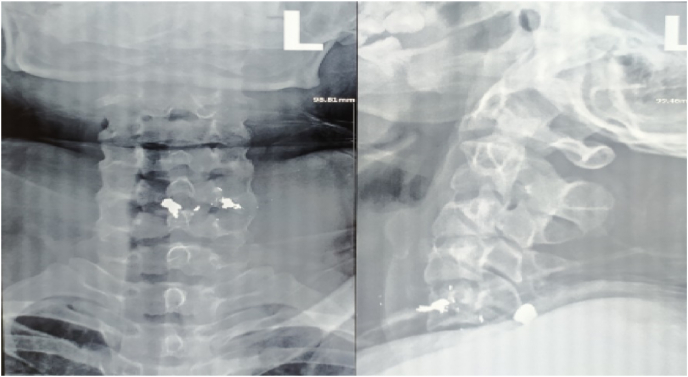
Initial plain cervical radiograph.
